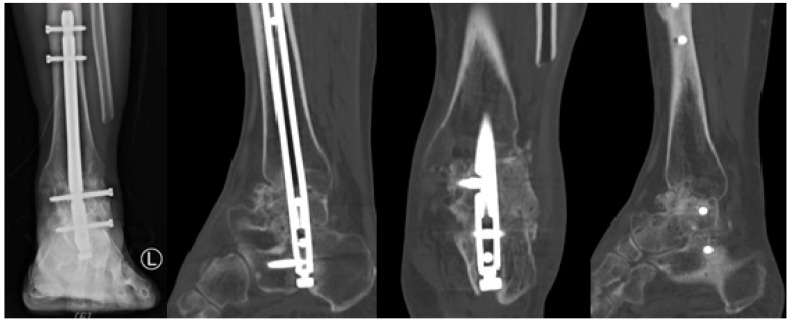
Two-Years Postoperative X-Ray and Computed Tomography Findings.
